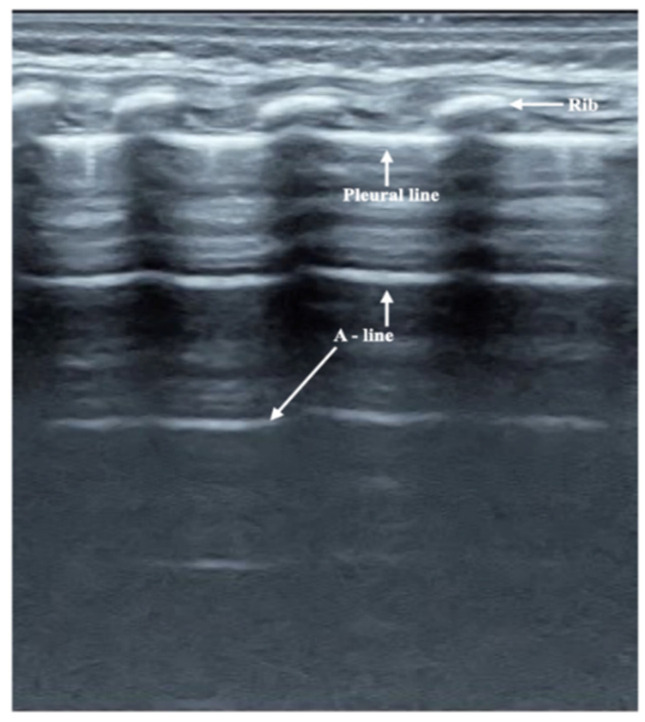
Normal Lung Ultrasound Manifestation. On B-mode ultrasound, the pleural line and A-line were parallel to each other, which formed a kind of bamboo-like ultrasound image, i.e., the bamboo sign.
